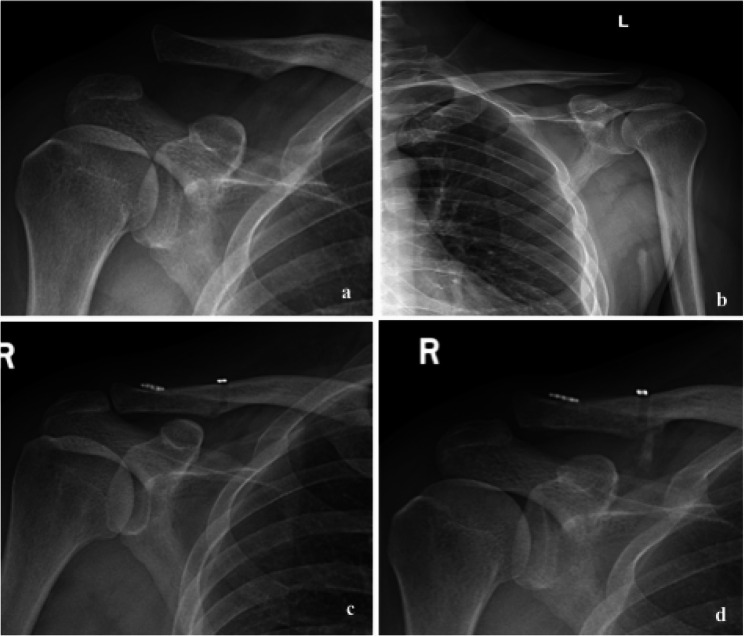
a A 46-year-old man with Rockwood type V AC joint dislocation. b X-ray film showed healthy X-ray film. c X-ray film showed a good reduction of the AC joint after surgery. d The operatively treated AC joint was slightly secondary displacement trend at 3 months postoperatively, and calcification of coracoclavicular ligament was observed
